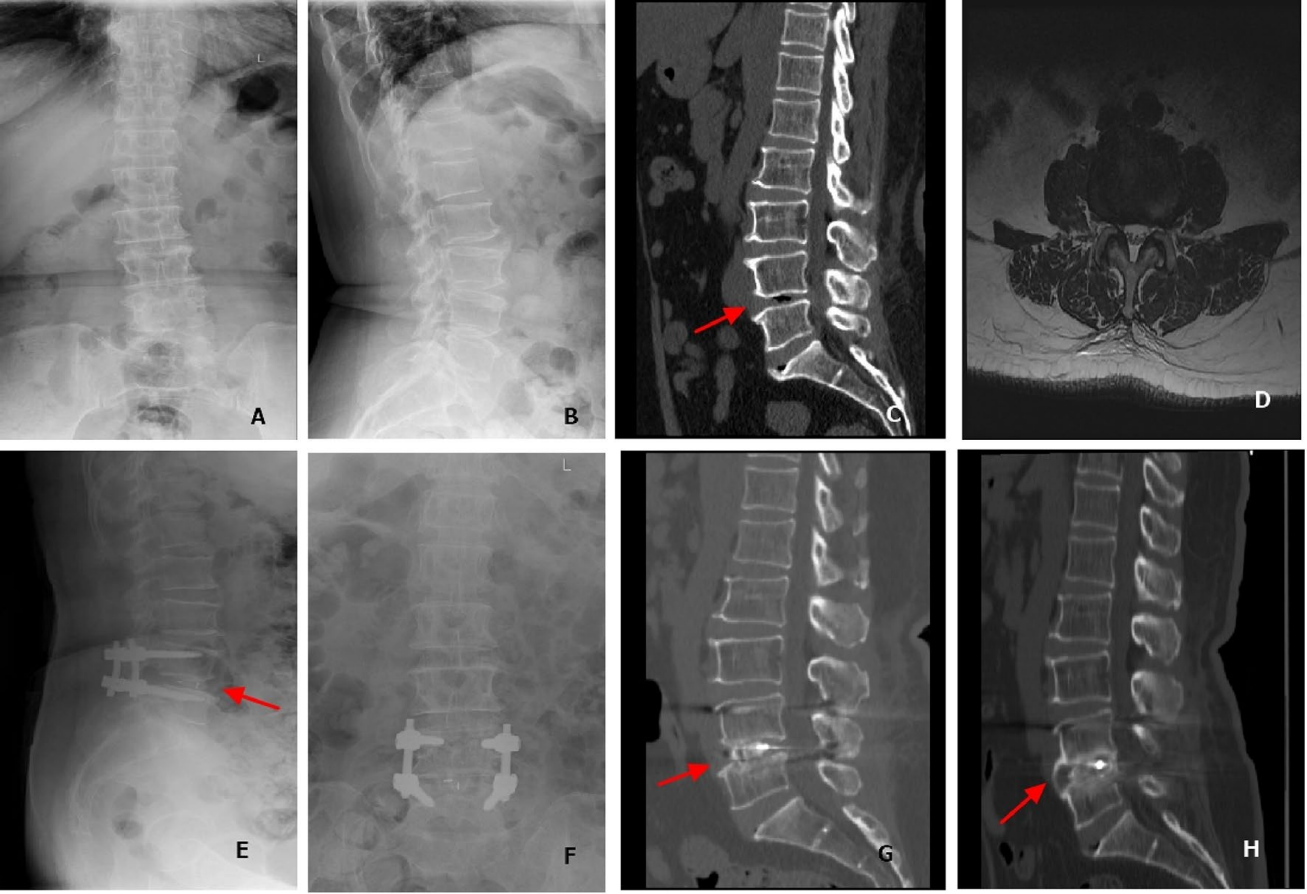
Typical case of TLIF, a 64-year-old female patient. On April 15, 2019, leg and back pain for 3 years, and DR (A, B), CT (C) and MR (D) were performed. After admission, L4-5 TLIF was performed, and DR was reexamined after surgery (E, F). 3 months later, examination of CT (G), 12 months later, examination of CT (H). Bone bridge growth ahead arrow sign.
